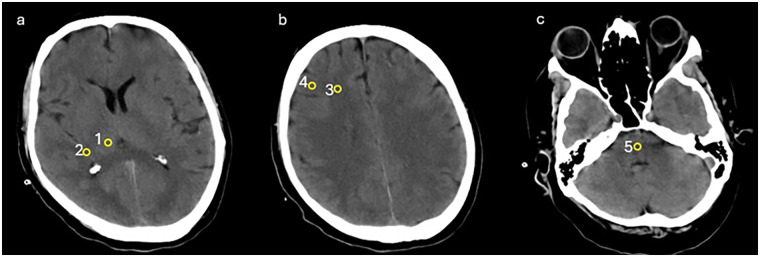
Axial CT image of a 65-year-old man showing the region of interest at the (a) basal ganglia level (BGL): 1—gray matter thalamus (GMT), 2—white matter posterior limb of the internal capsule (WMPIC); (b) centrum semiovale level (CSL): 3—adjacent cortical gray matter (ACGM), 4—frontal white matter; and (c) 5—pons.
